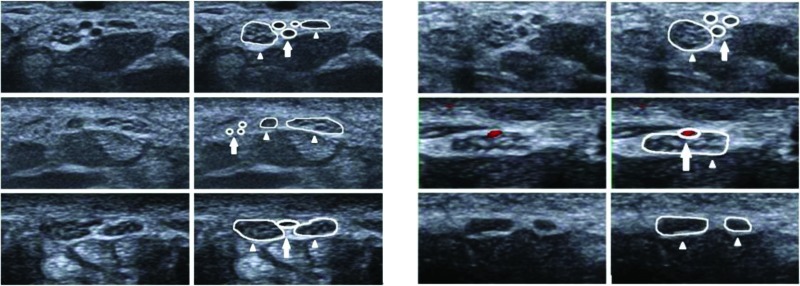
Transverse ultrasonographic image (left side) and corresponding outline-marked image (right side) of a bifid median nerve (thin arrow) and PMA (thick arrow) at the level of the proximal carpal tunnel. Different PMA positions in relation to the median anatomy and a bifid median nerve with an intermediate position in relation to the PMA were most frequently observed.
